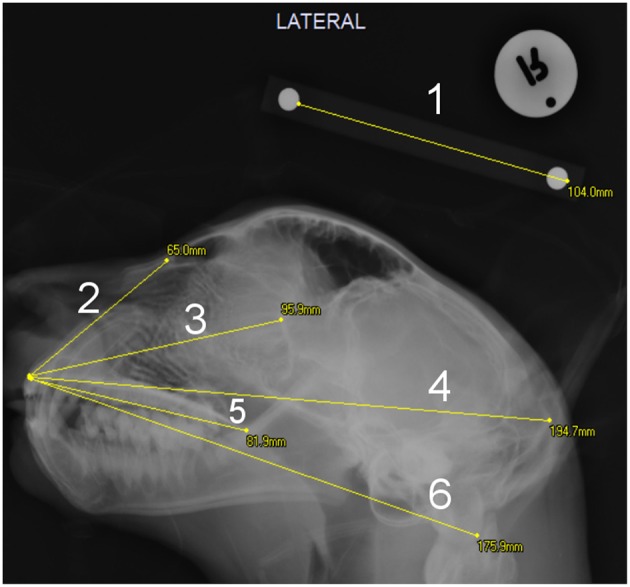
Extent of measurements on the lateral skull radiographs: 1- The 100 mm scale barr; 2- Facial length2; 3- Facial length1; 4- Total skull length; 5- Palate length; 6- Condylobasal length.
